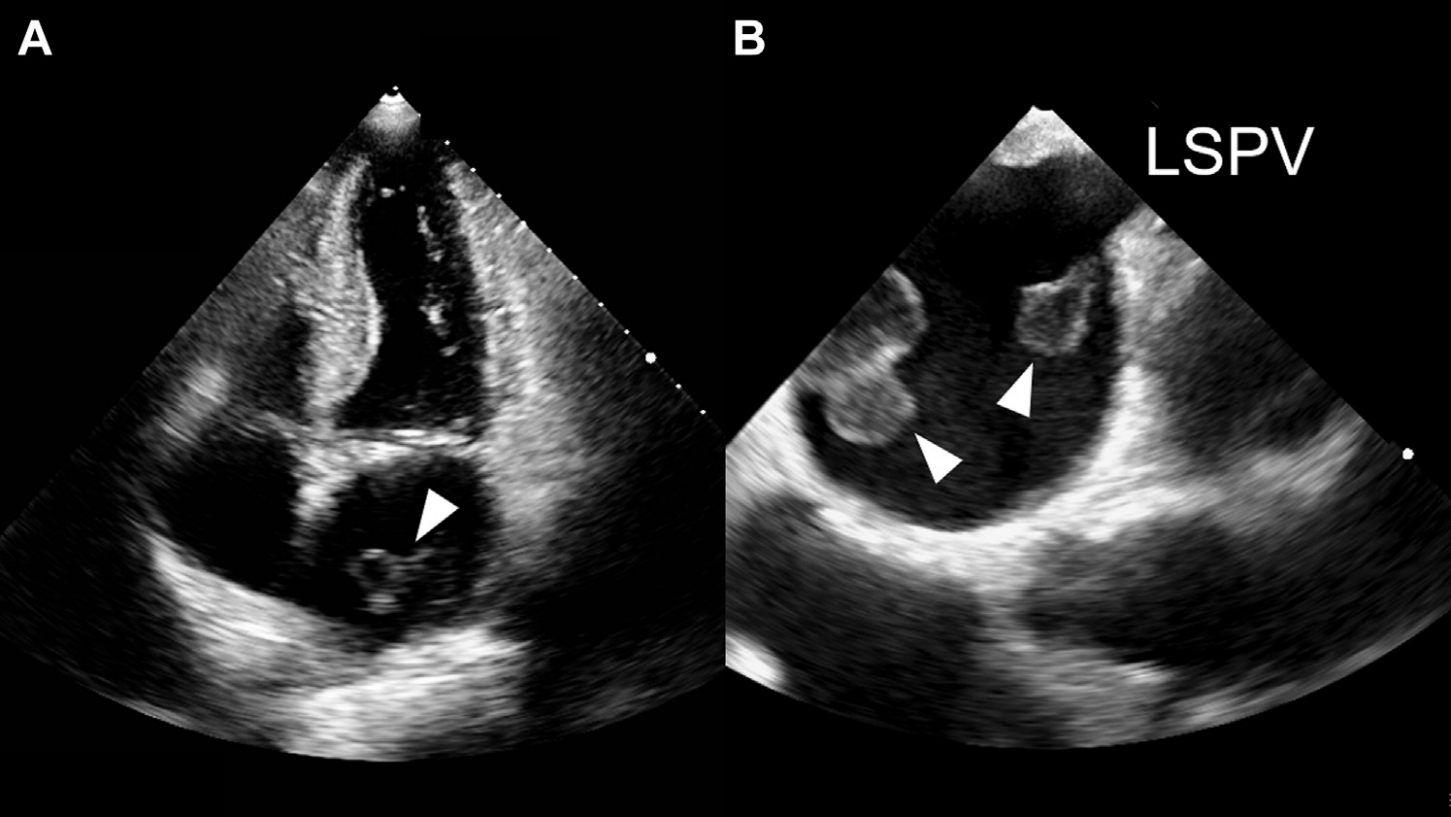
Giant thrombi in the left atrium visualized by transthoracic and transesophageal echocardiography. A: Transthoracic echocardiography detected large, abnormal structures with beaded appearance in the left atrium (*arrowhead*). The left atrium was dilated, but the other findings were not significant. B: Transesophageal echocardiography revealed de novo pedunculated giant mobile masses, which were attached to the ostia of the left superior and right inferior pulmonary veins (*arrowhead*). The surfaces of these masses were partially collapsed. Thrombus in the left atrial appendage and spontaneous echo contrast were not detected. LSPV = left superior pulmonary vein.
